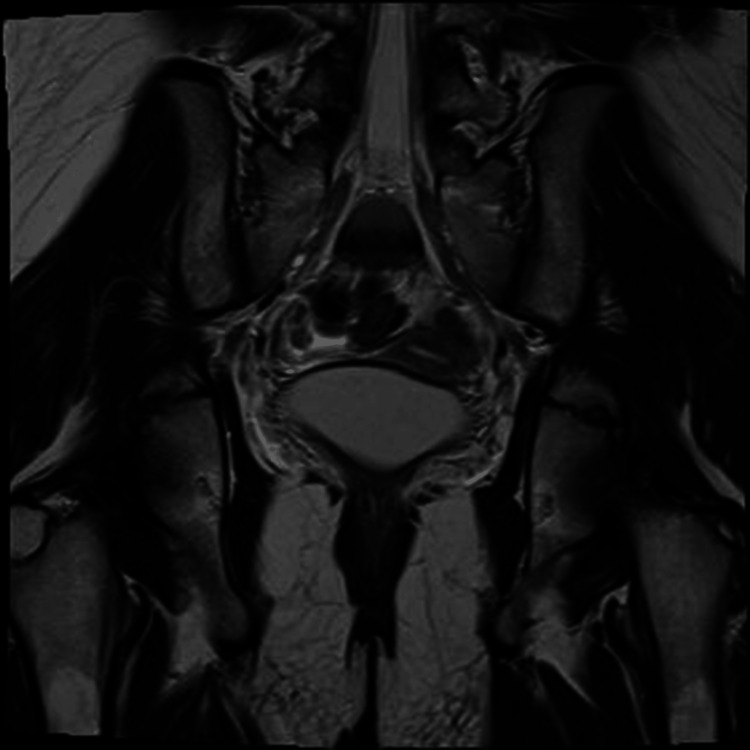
Magnetic resonance imaging (MRI) of the abdomen showing an absent uterus.
